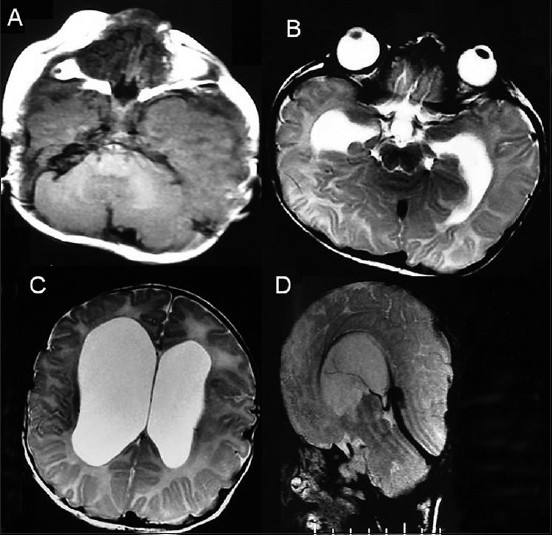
Case 2: (A–D) Magnetic resonance images showing gross ventriculomegaly with periventricular lucency and a chinked fourth ventricle. The sagittal image showed a steep angulation of the tent and straight sinus and a brachycephalic head
